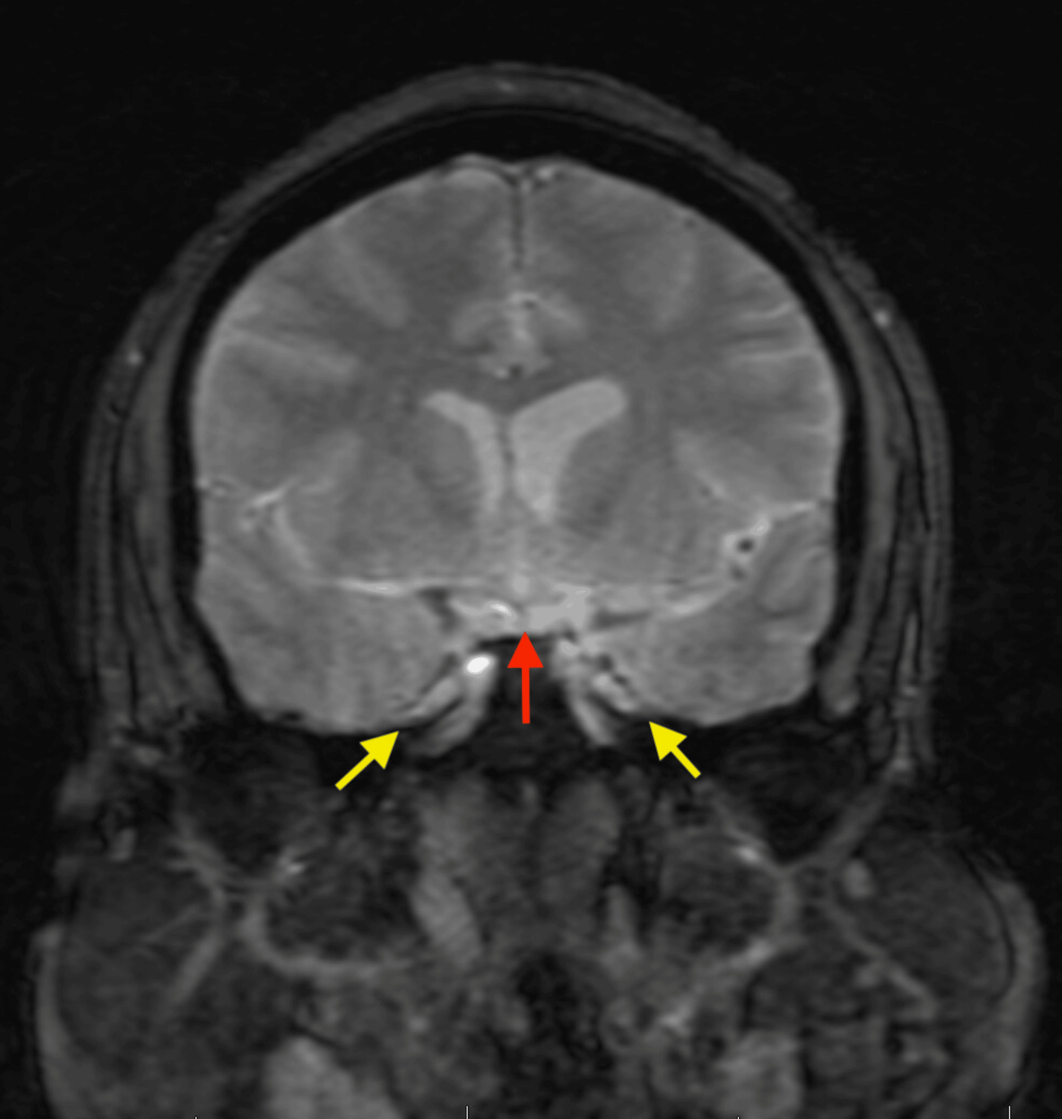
Non-contrast MRI brain demonstrating findings suggestive of elevated intracranial pressure. Multiplanar T2-weighted sequences show symmetrically prominent Meckel’s caves (yellow arrows) with mild flattening of the superior pituitary margin (red arrow), changes associated with chronically raised intracranial pressure.
